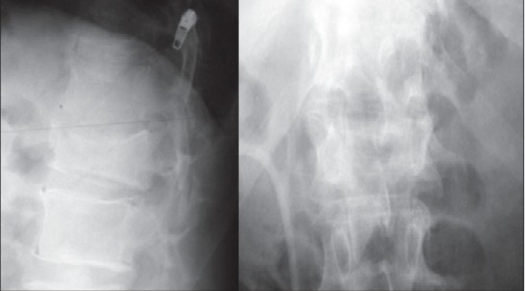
X-ray lumbar spine (AP and Lateral) in a 48-year-old male patient showing a burst fracture of L_2_
